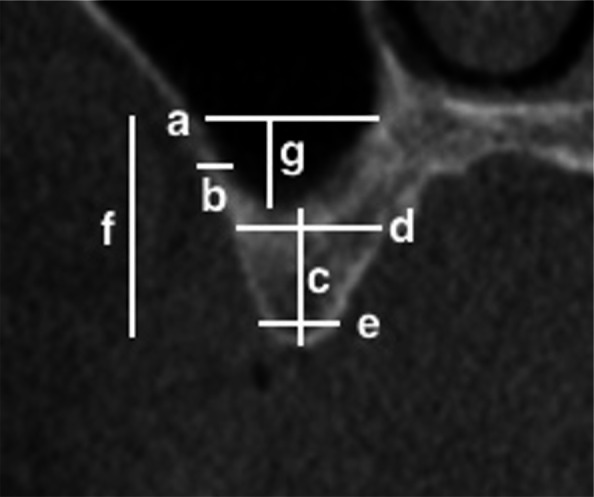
Explanation of linear measurements in CBCT considered in the study. (a) AAA; (b) Thickness of the lateral sinus wall; (c) Height of the residual alveolar ridge; (d) Width of the residual alveolar ridge (basal level); (e) Width of the residual alveolar ridge (crestal level); (f) Distance from AAA to the alveolar crest, (g) Distance from the AAA to the sinus floor.
